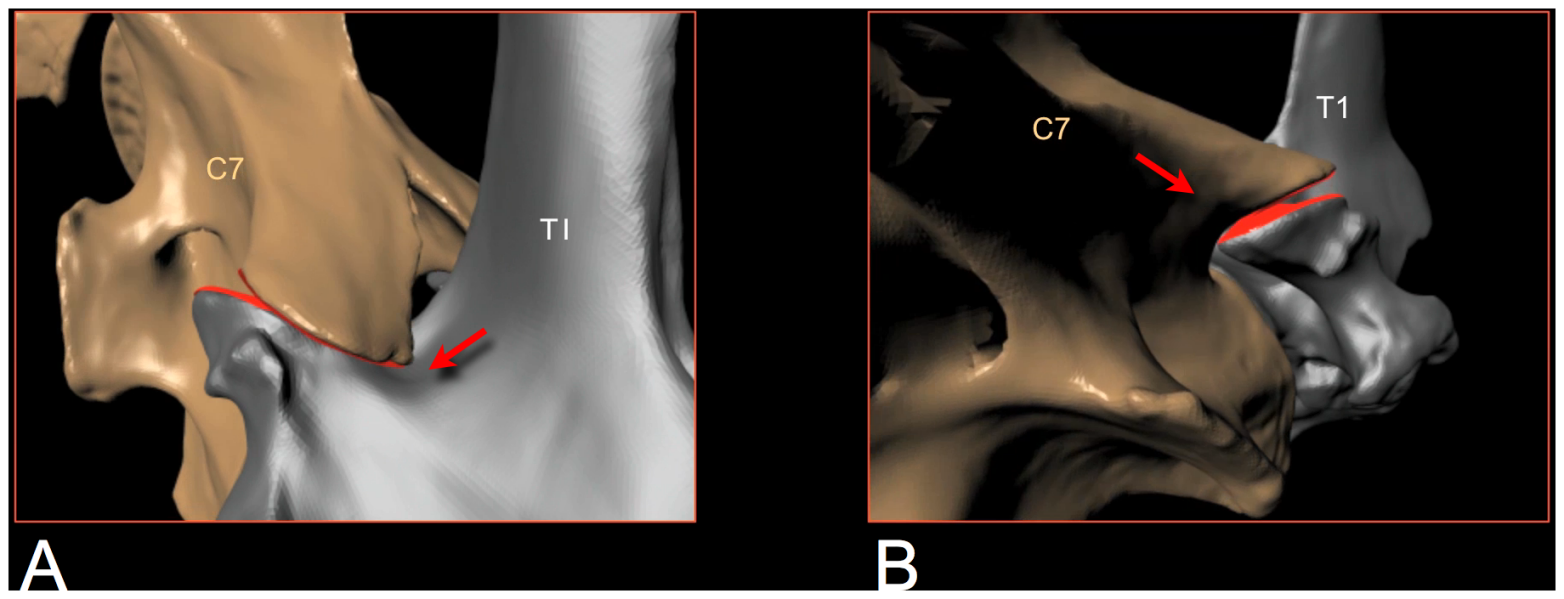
Bracing at the base of the giraffe's neck. The base of the giraffe's neck is braced to protect the intervertebral joints from excessive strain on their synovial capsules and to rigidify the neck as it reaches the limits of range of motion. As the neck is raised at the base (A), the postzygapophyses of C7 travel posteriorly until they wedge into depressions in the neural spines of T1 just behind the prezygapophyses (see arrow). Another bracing scheme applies when the neck is deflected laterally (B), In defecting the neck to the left, for example, C7 bears against the left postzygapophysis of T1, see arrow. In either dorsal or lateral flexion the two vertebrae progressively lock up firmly and stably. At these extremes the zygapophyses maintain substantial overlap (roughly a ZSF of roughly 0.5). CT data provided courtesy American Museum of Natural History and Timothy Rowe, University of Texas. Supplemental material: Movie S2, Movie S3.
